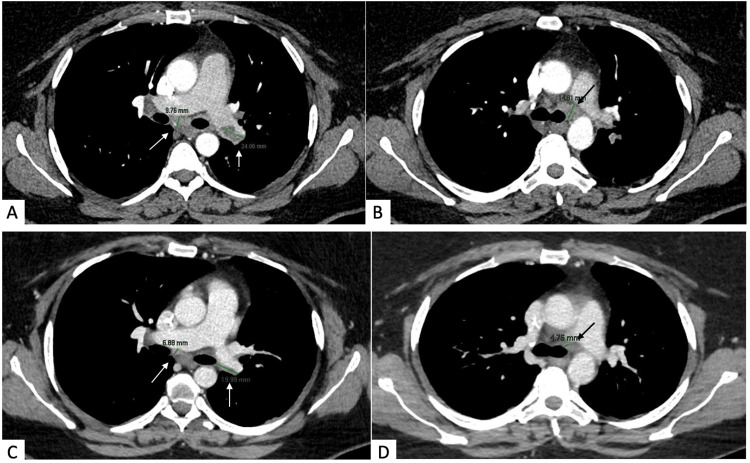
Comparison of pre- and post-treatment CT scans Basal CT scan (January 2020): Image A shows a lesion of 24 mm in the left pulmonary artery (T1, 24 mm) and subcarinal nodes (T3, 10 mm); image B shows the paratracheal left lower nodes (T2, 15 mm). CT scan performed on May 2020: Image C shows the T1 (20 mm) and T3 lesions (5 mm); image D shows the T2 lesion (5 mm).
